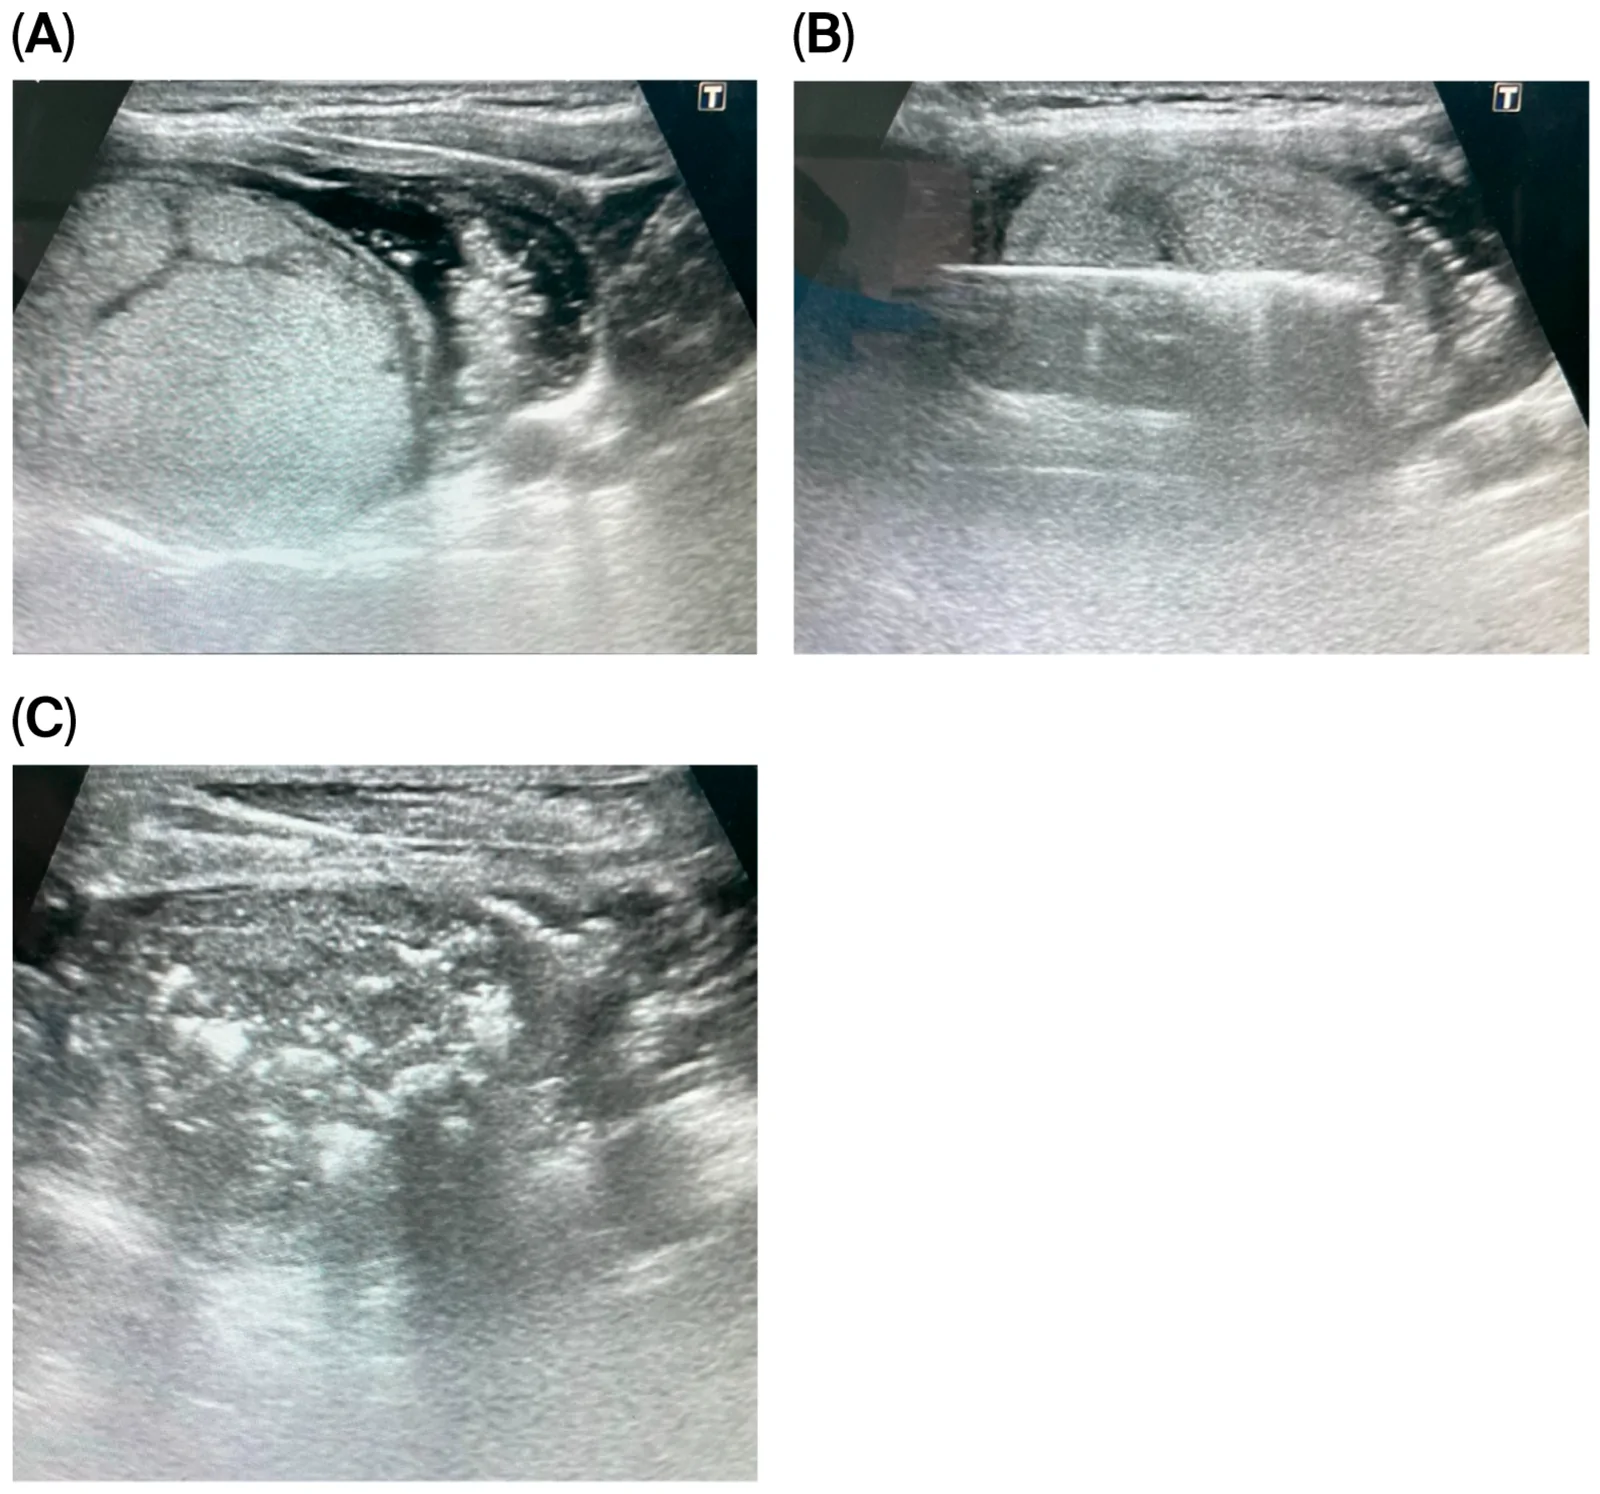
(A) Ultrasound-guided hydrodissection creating a protective fluid barrier between the thyroid nodule and adjacent critical structures. (B) Hyperechoic microbubbles generated during microwave ablation of the thyroid nodule. (C) Post-ablation ultrasound image demonstrating the ablation zone within the treated thyroid nodule.
